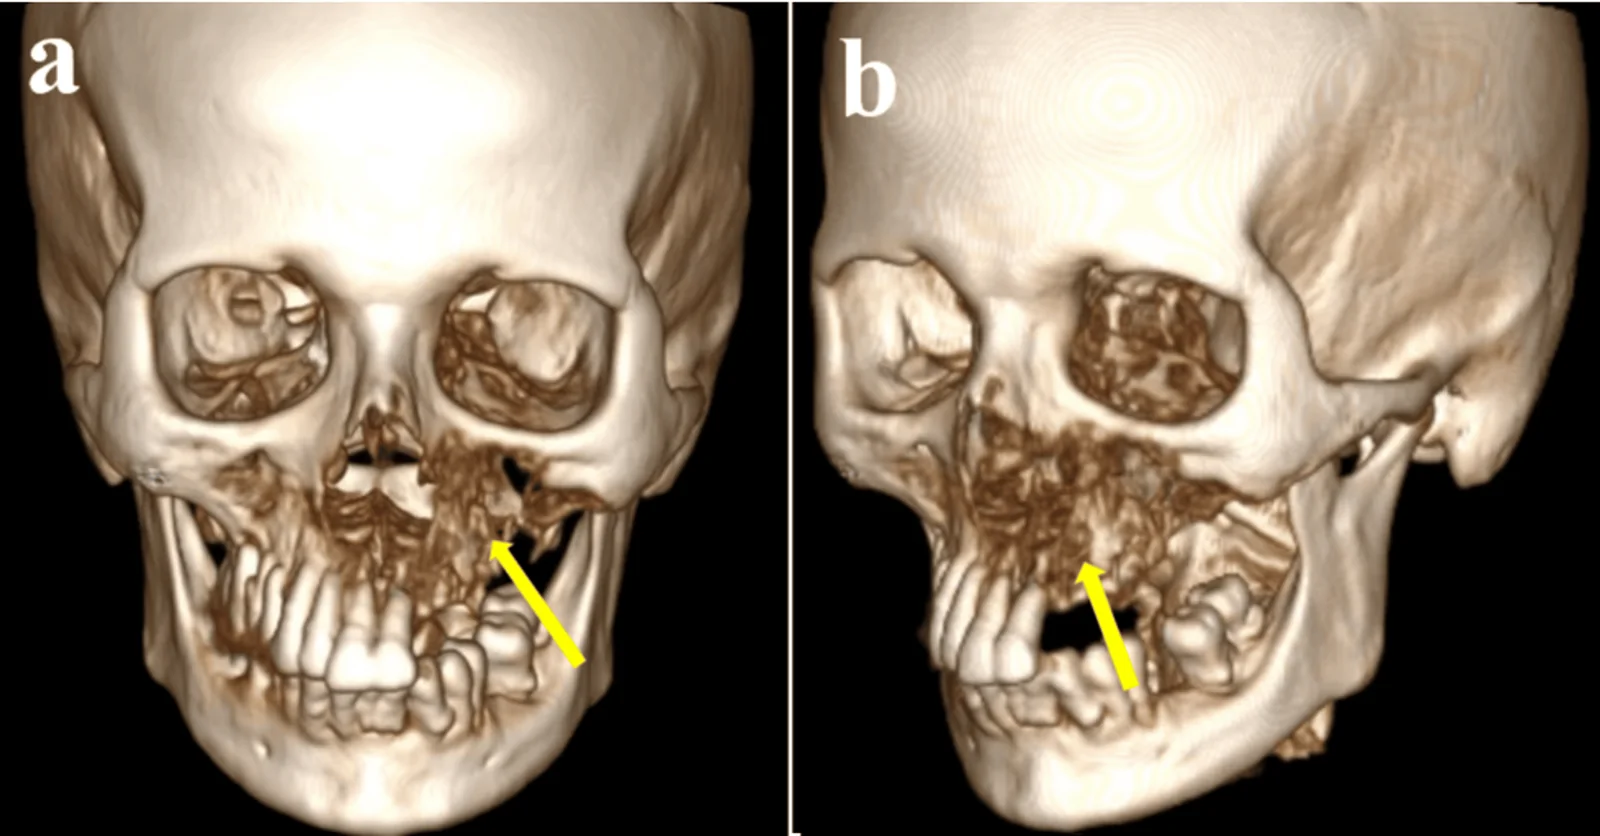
Three-dimensional reconstructed CT images of the facial skeleton. (a) Frontal view and (b) left anterolateral oblique view show extensive osteolytic destruction involving the left maxillary alveolar process, hard palate, anterior wall of the maxillary sinus, piriform aperture, and zygomaticomaxillary buttress (yellow arrow), resulting in marked loss of the left midfacial bony framework. CT: computed tomography.
